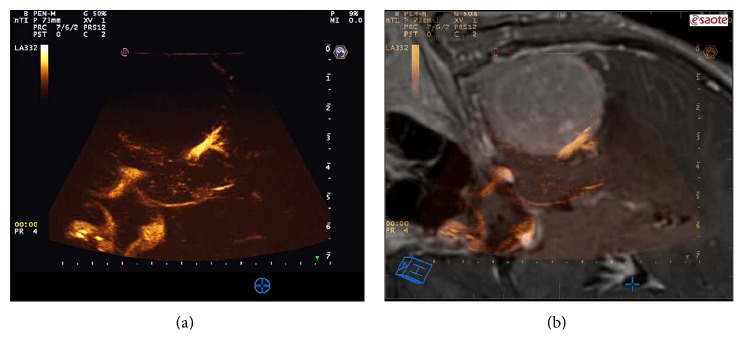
CEUS imaging. In the left box, CEUS scan of the lesion is displayed; in the right box, fusion imaging between intraoperative CEUS and corresponding preoperative MRI aid in US interpretation. Because the main vascular supply of this lesion was from dural attachment, once it was coagulated, no perfusion of the lesion was noticeable. The principal vascular structures are clearly visible: plexus of Willis, basilar tip, cavernous sinus, and the neighbor middle cerebral artery.
